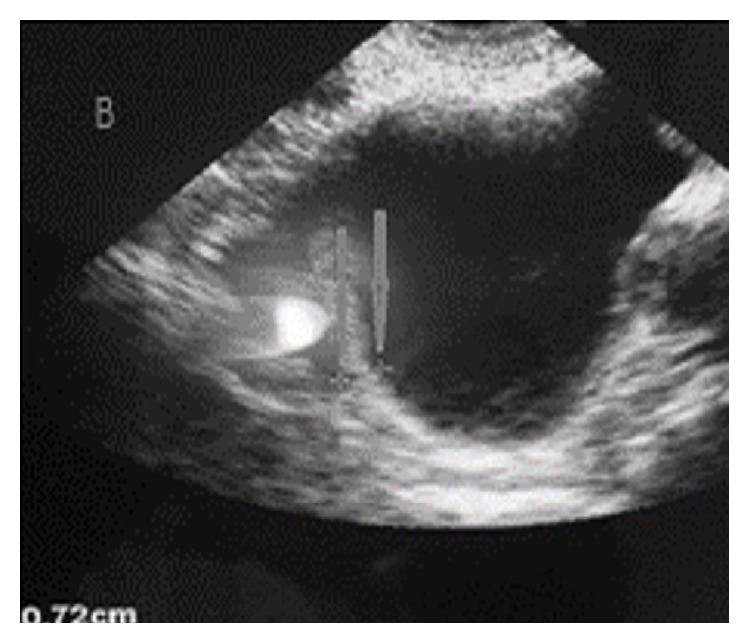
B mode ultrasound of the bladder showing a thickened bladder wall (arrows).
